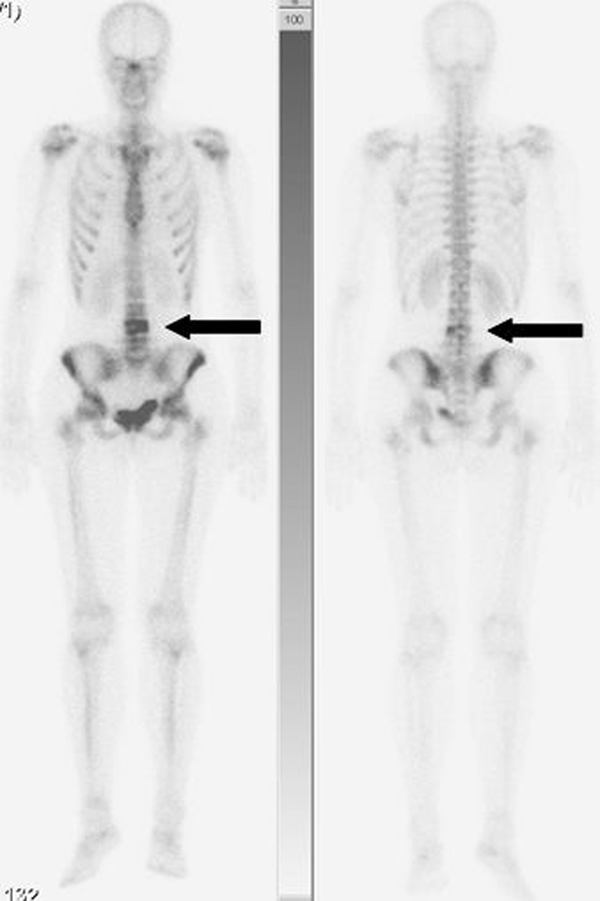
Whole-body bone scintigraphy with 99mTc-HDP showing an intense, local enhanced uptake at level L4 with areas of decreased uptake demonstrating the cystic components.
